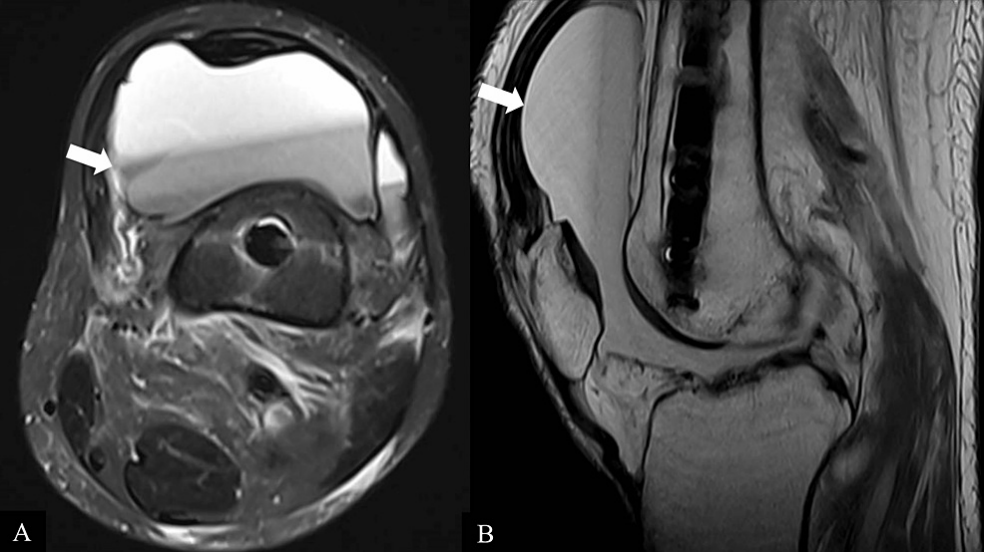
Axial (A) and sagittal (B) views of the left knee on magnetic resonance imaging (MRI) showing a large joint effusion with a fluid-fluid level, demonstrating largely T2-weighted hyperintense signal and intermediate T1-weighted signal suspicious for hemarthrosis.
